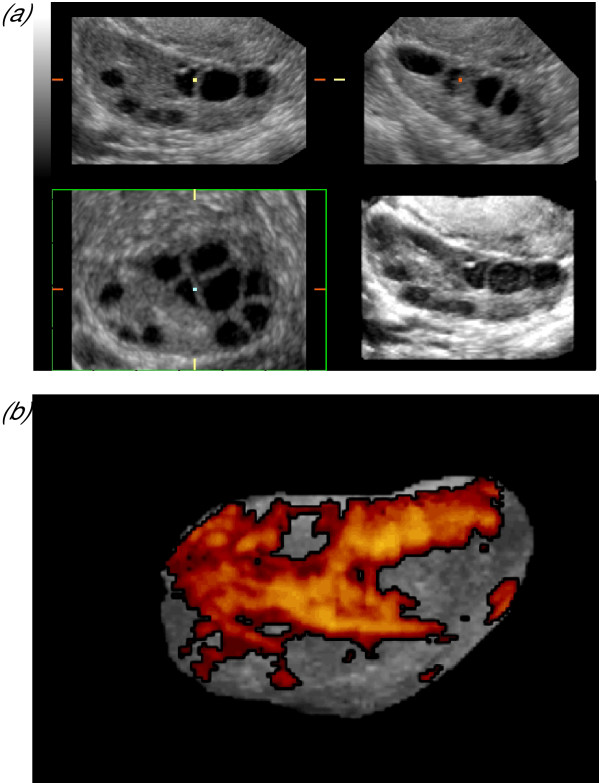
Polycystic ovaries*. Three-dimensional sonography facilitates objective assessment of the ovarian stroma, through measurement of its mean grey signal intensity, its vascularity and its volume, which may be calculated by subtracting total follicular volume from total ovarian volume (Fig. 9A). Ovarian blood flow is increased and associated with significantly higher three-dimensional indices of vascularity than ovaries with a normal appearance (Fig. 10b).
